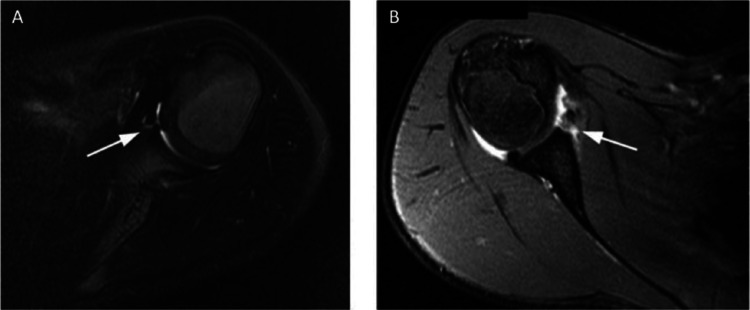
(A) Postreduction axial T2-weighted MRI of the shoulder in a 9-year-old boy who had an anterior glenohumeral dislocation. The anteroinferior capsulolabral tissue (arrow) was intact, with no evidence of a Bankart lesion in all views. (B) Postreduction axial T2-weighted MRI of the shoulder demonstrating a bony Bankart lesion (arrow) in a 14-year-old boy with an anterior glenohumeral dislocation. Figure taken from Li et al. 2013 [36]. Reprinted with permission from Wolters Kluwer Health, Inc [36]
